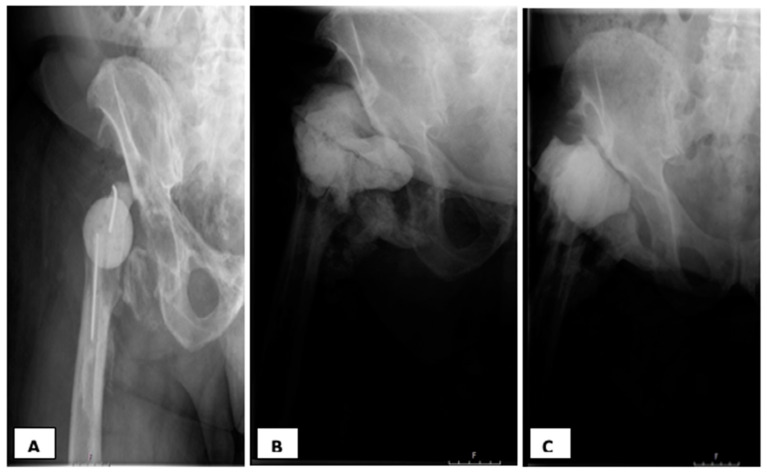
Hip radiographs: periprosthetic joint infection (PJI) with history of three prior debridement surgeries and systemic antibiotics. (A) First Hip spacer (Girdlestone); (B) Status post second spacer exchange (third spacer) for persistent infection; (C) Status post third spacer exchange: failure of intended reimplantation. Clinical history: First exchange occurred after recurrence of fever, elevated CRP, local signs of hip infection and positive urinalysis s/p two-weeks off antibiotics. Of note, purulence was observed during the first exchange surgery. Second Exchange occurred after a purulent drainage, with an aspiration culture growing methicillin-resistant *Staphylococcus aureus* (MRSA). After a third spacer exchange, the intra-operative cultures grew Klebsiella Pneumoniae.
